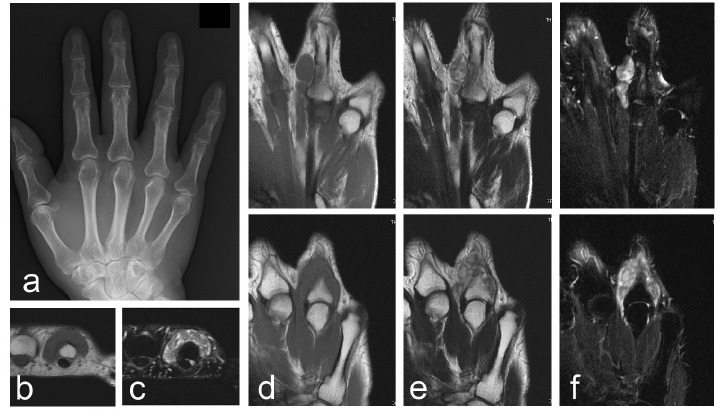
Images of a 66-year-old male with extra-articular tenosynovial chondromatosis of the right ring finger. Plain radiographs of the hand showed no abnormality (a). An MRI showing multinodular lesions with homogeneous low signal intensity on the T1-weighted images (b and d) and heterogeneous low to high signal intensity on the T2-weighted images (e) on the dorsal side of the proximal phalanx. Fat suppression on the T2-weighted images demonstrates an extension of the lesion (c and f). The top and bottom images of panel d-f are sequential.
